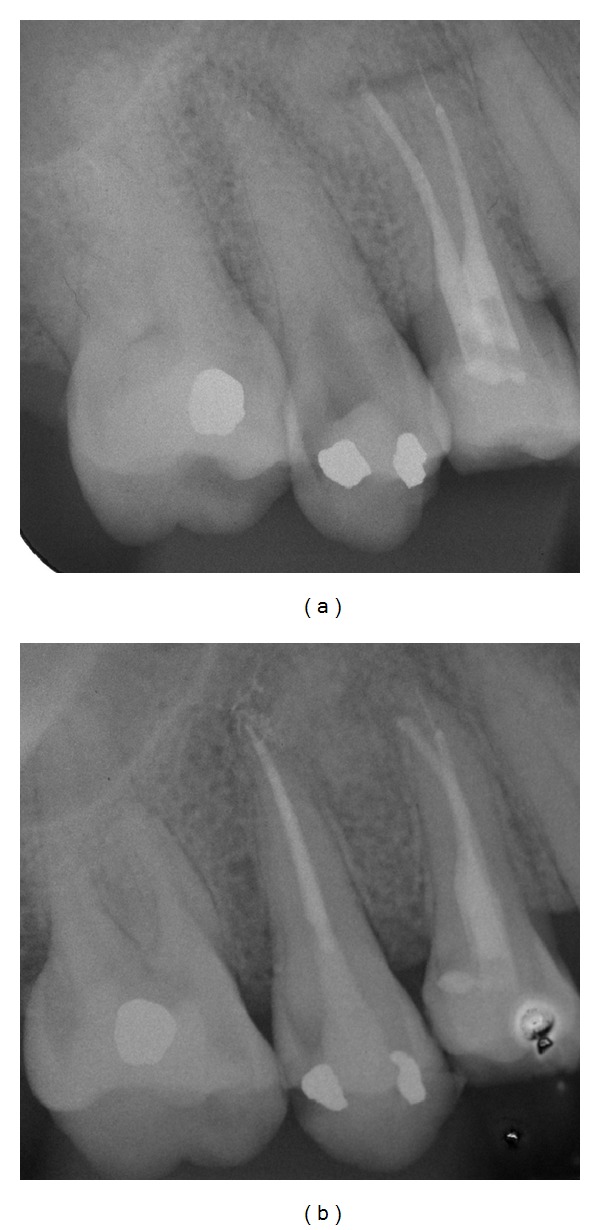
(a) Initial radiograph showing constricted canals. (b) Final radiograph.
